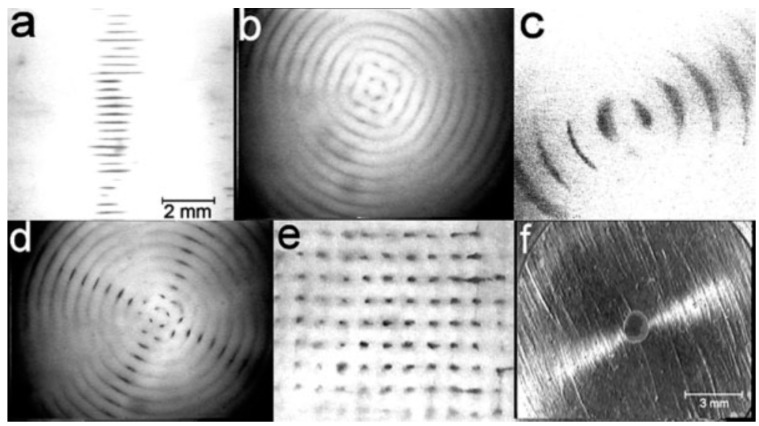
Cell aggregation under ultrasound standing wave and view normal to sound propagation (a) column of discoid aggregates in a plane cylindrical resonator; (b) initial pattern in tubular resonator-concentric cylinders; (c) aggregates of different sizes in a tubular resonator; (d) in plane and tubular resonator; (e) in a pair of two transducers perpendicular to each other; and (f) view in the direction of sound propagation of 3D RBC aggregate in the center of half-wavelength resonator, courtesy of [71].
